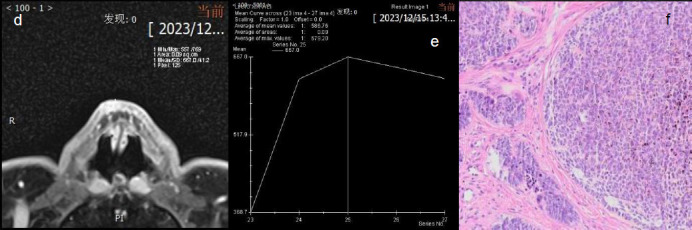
MRI of BCC in nasal tip. The tumor appeared isointense on T1WI (a), hyperintense on T2WI (b) and FST2WI (c). The dynamic enhancement images showed significant enhancement (d) with outflow pattern in dynamic enhancement curve (e). The pathology confirmed the tumor was BCC (10×) (f).
